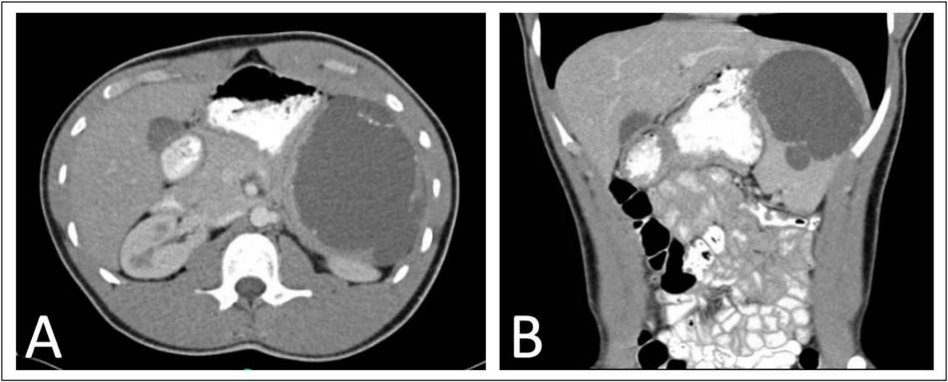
Preoperative (A) axial and (B) coronal abdominal CT images showing complex cystic splenic mass.
